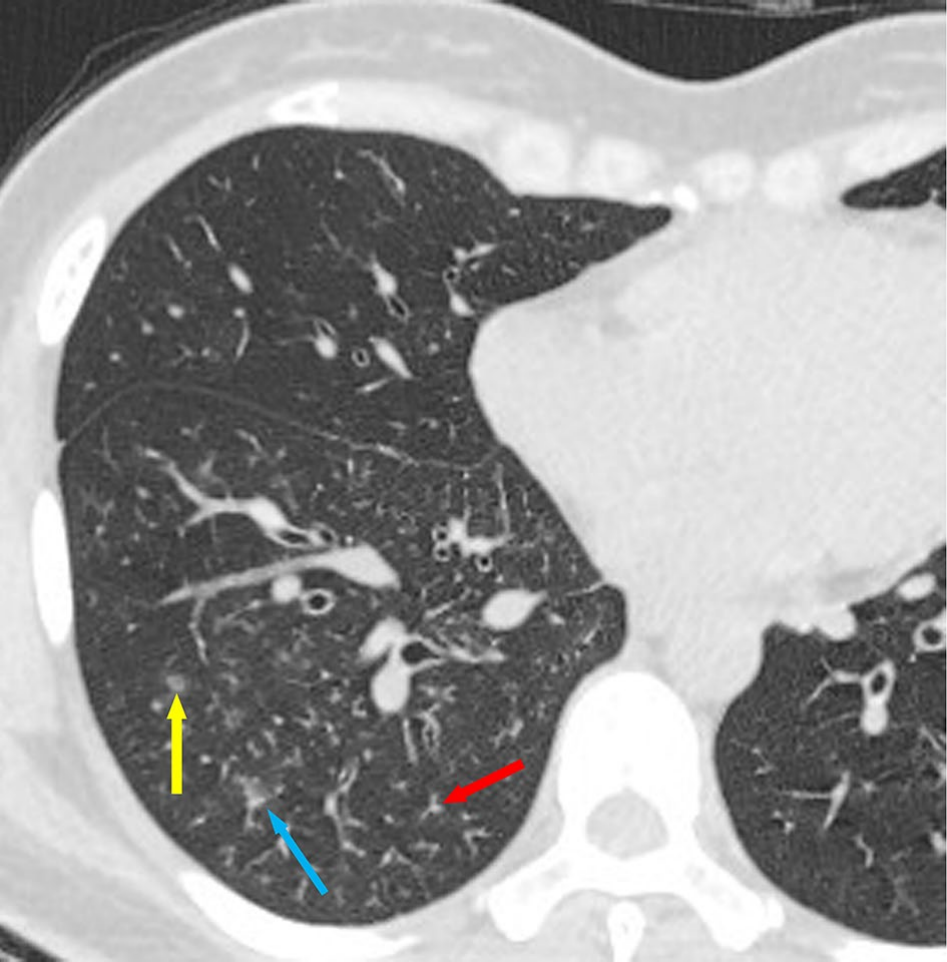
HRCT findings of a 30-year-old female case with mycoplasma pneumoniae pneumonia. The red arrow showed the tree-in-bud sign (TIB). The yellow arrow showed the ground-glass opacity (GGO). The blue arrow showed the tree fog sign (TIB + GGO).
